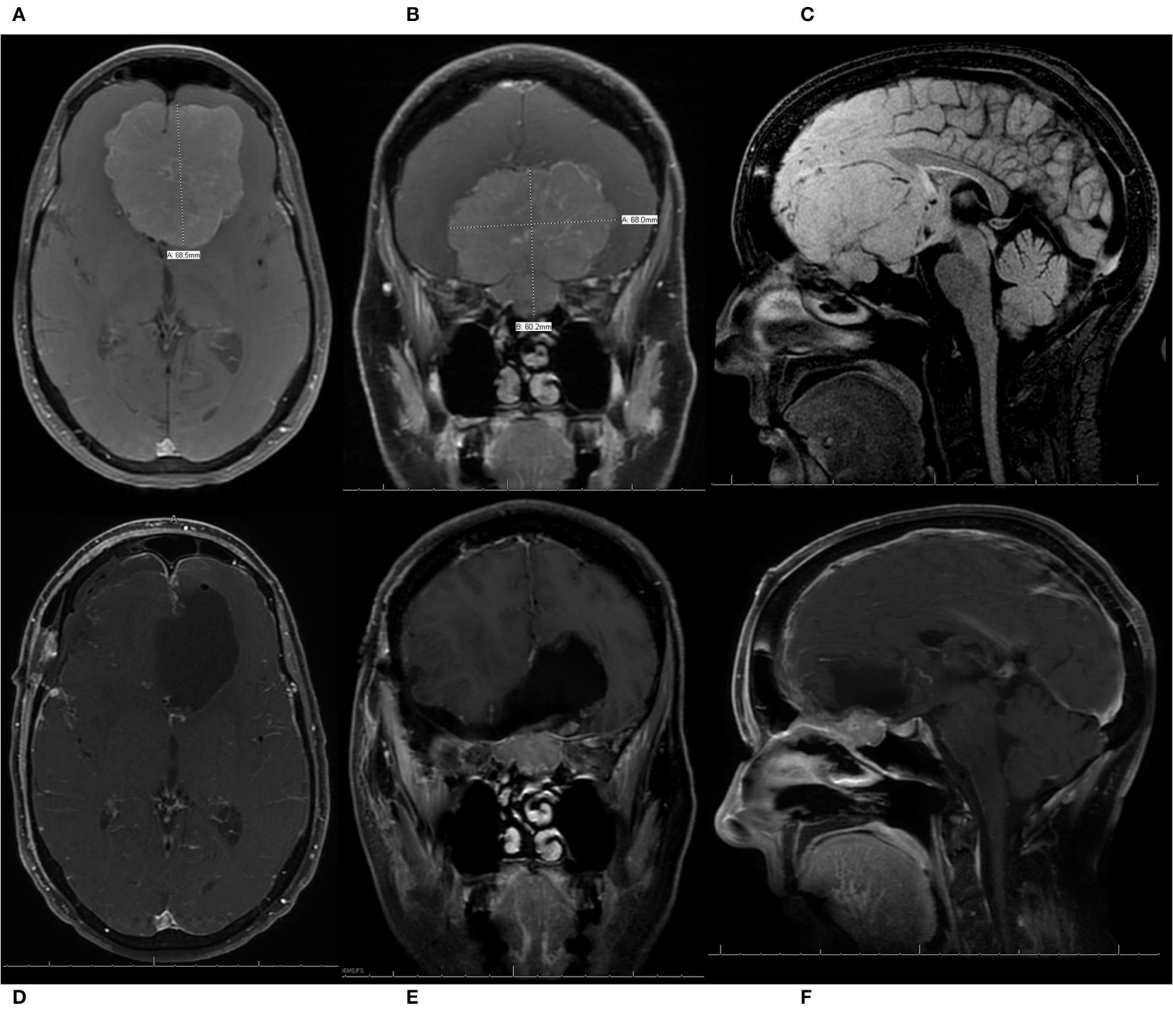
A patient presented with progressive vision loss, anosmia, gait instability, and cognitive decline and was found to have a 6.8 cm olfactory groove meningioma (OGM) with expansion into the endonasal cavity. The internal carotid arteries and their branches were pushed posteriorly by the lesion. The patient underwent a pterional craniotomy for resection of the large WHO grade I OGM with a residual tumor left in the endonasal compartment. The patient had an immediate improvement in vision, with no new neurologic deficits, and was discharged home from the hospital on post-operative day 5. She also enjoyed recovery of taste/smell and gradual but full recovery of her cognition. (A) Pre-operative axial T1 MRI with contrast demonstrating a large 6.8-cm OGM with the anterior cerebral arteries (ACAs) pushed posteriorly. (B) Pre-operative coronal T1 MRI with contrast, demonstrating a large OGM with extension through the cribriform plate into the endonasal cavity. (C) Pre-operative sagittal T1 MRI without contrast, demonstrating a large OGM with endonasal extension and displacement of the ACAs posteriorly. (D–F) Post-operative axial, coronal, and sagittal T1 MRIs with contrast, respectively, demonstrating resection of the intracranial component of the large OGM, with preservation of the ACA vasculature and a residual meningioma left in the endonasal compartment to prevent the development of a cerebrospinal fluid leak.
